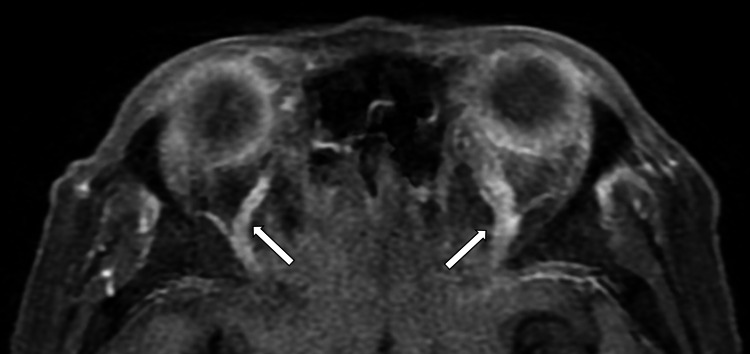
Axial T1-weighted (fat suppression) post-contrasted MRI orbit image. MRI orbit image showed the dilated left and right superior ophthalmic veins (white arrows). The bilateral superior ophthalmic veins were opacified by contrast with no filling defect seen within.
